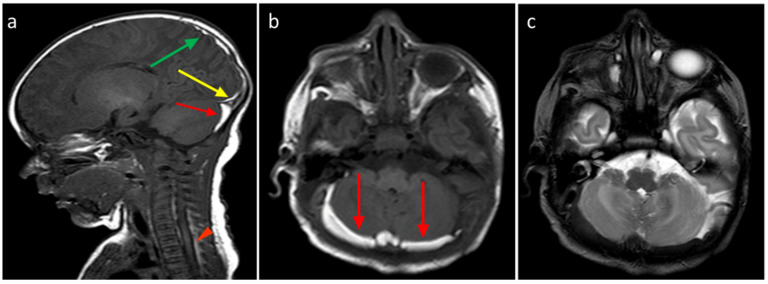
(a) Birth-related SDH in a 2-day-old infant born spontaneously. Right paramedian sagittal plane SE T1-weighted sequence—panoramic view of multiple subdural hematomas showing occipital supratentorial (yellow arrow) and parietal (green arrow) and cerebellar infratentorial (red arrow) distribution but also in the spinal canal anteriorly and posteriorly in the cervico-dorsal tract (arrowheads). (b) Axial T1-weighted SE sequence passing through the posterior cranial fossa—the infratentorial hematoma shows a development posterior to the vermis and the cerebellar hemispheres, with a greater thickness on the right (arrows). (c) FSE T2-weighted sequence on the axial plane at the same level as (b) (b)—the hematoma shows a hypointense signal, less easily appreciable (arrows), but which, in relation to the hyperintense appearance in T1, allows us to date the hematoma.
